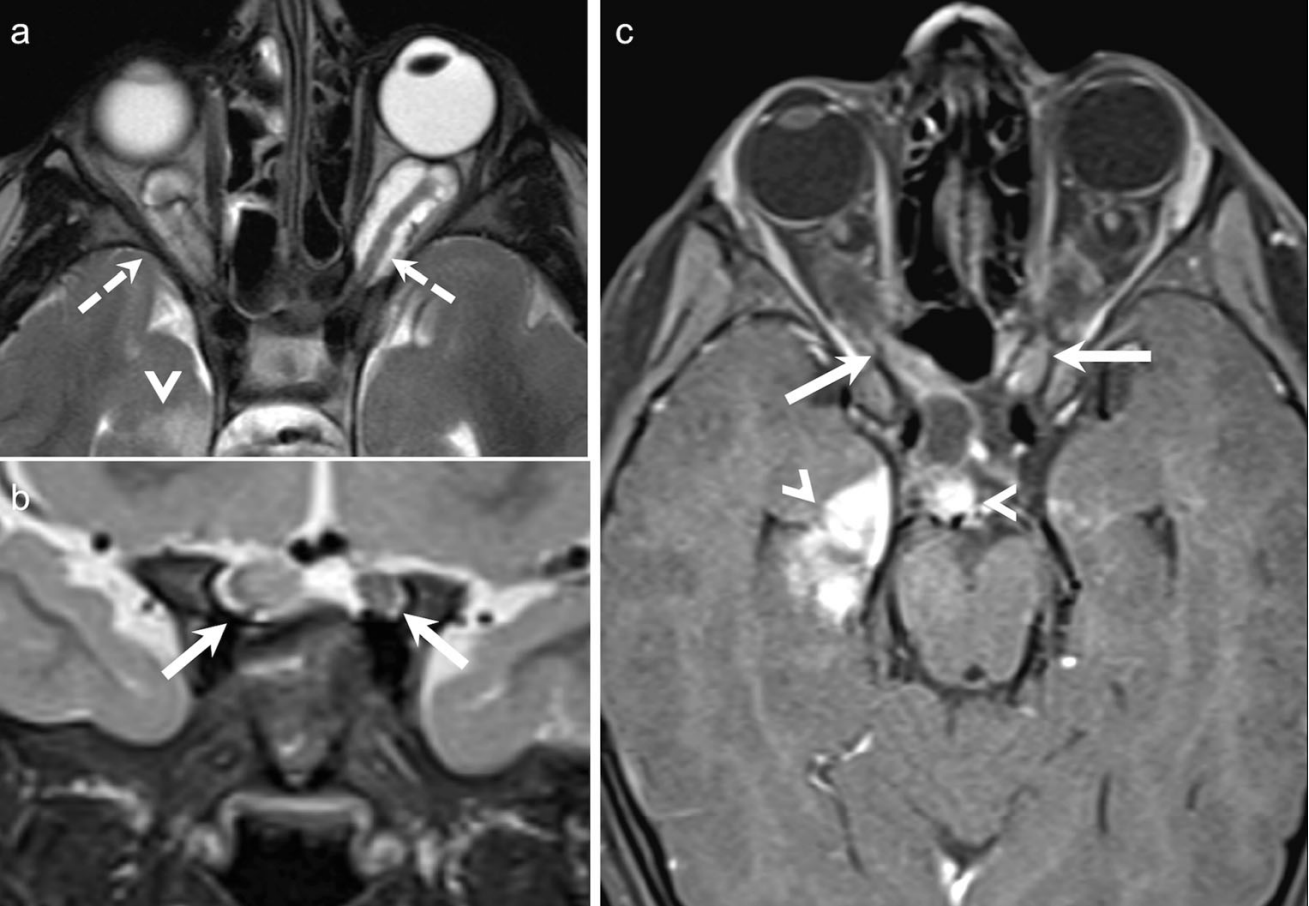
5-year-old boy with NF-I. a. Axial T2W MR image of the orbits shows bilateral ONG (dashed arrows) causing fusiform enlargement and kinking of the optic nerves. A focal T2- hyperintense lesion (arrowhead) is seen in the right mesial temporal lobe. b. Coronal T2WMR image of the same patient shows extension of the bilateral ONG along the intracranial segments of the optic nerves (arrows). c. Axial contrast-enhanced FS TIW MR image of the same patient shows prominent enhancement in the intracanalicular and intracranial segments of bilateral ONG (arrows). There is also avid enhancement in the suprasellar/chiasmatic region (arrowhead) and in the right mesial temporal lesion (arrowhead) in keeping with tumour extension along the optic chiasm and right optic radiation
